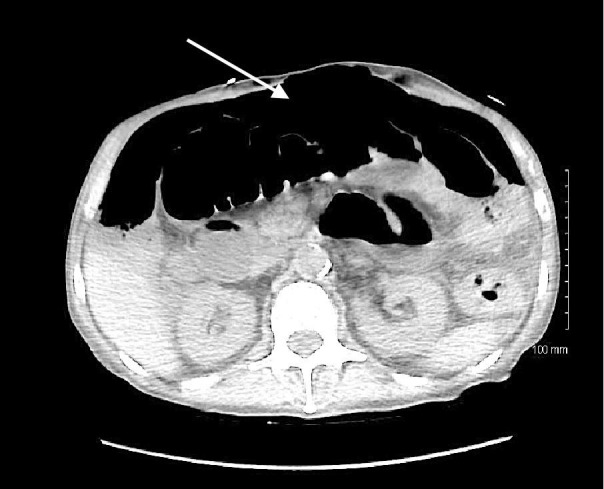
Computed tomography (CT) abdomen demonstrating large free intraperitoneal air (arrow) consistent with bowel perforation.
